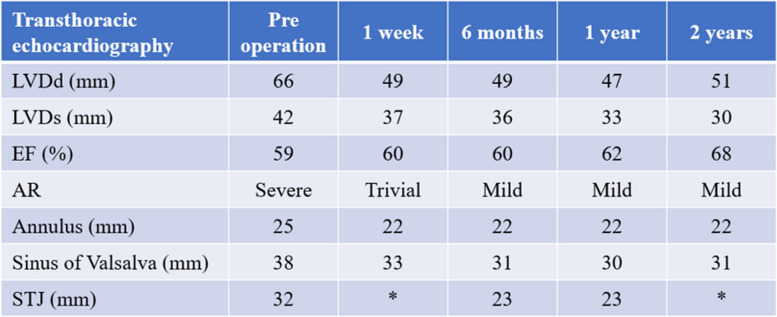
Results in transthoracic echocardiography
